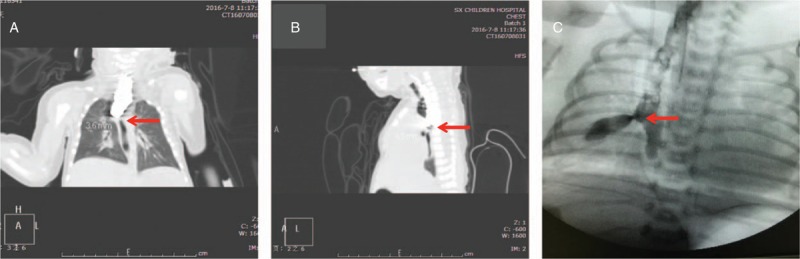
Picture from Case 2. A, Esophageal radiography (arrow indicating the esophageal blind end). B, CT sagittal plane (arrow indicating the distance between the 2 esophageal blind ends). C, Esophageal radiography 2 weeks after esophageal anastomosis (arrow indicating an abnormal esophagobronchial passage). CT = computed tomography.
